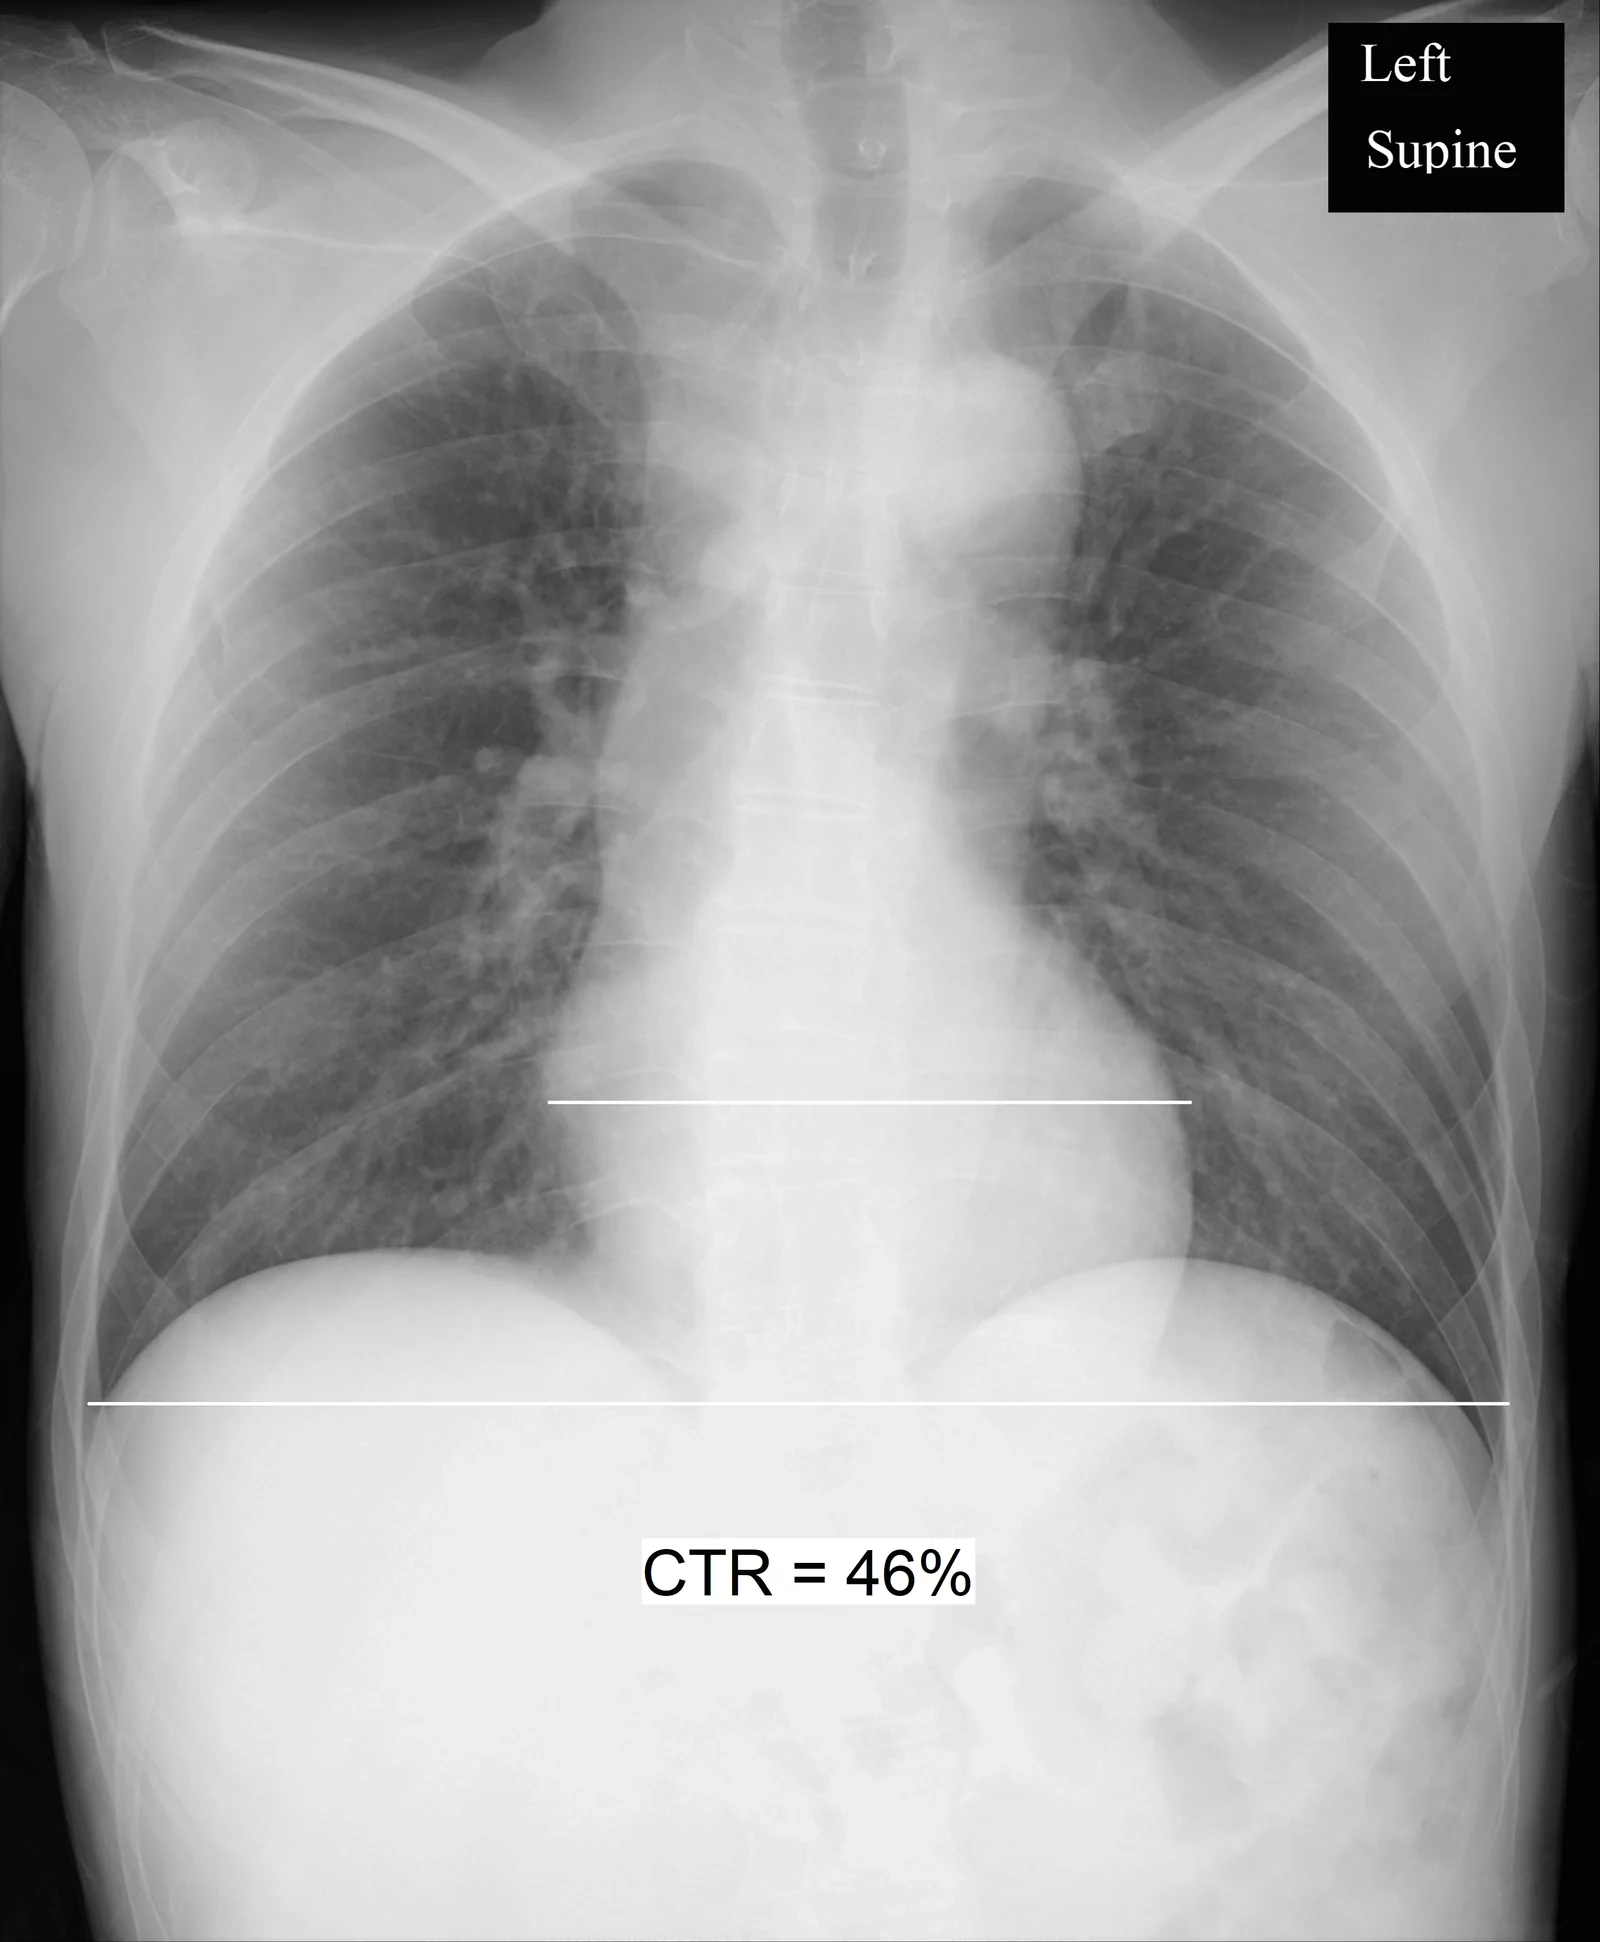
Chest radiograph on admission CTR: cardiothoracic ratio Chest radiography showed a CTR of 46% without pulmonary congestion, along with widening of the mediastinum
